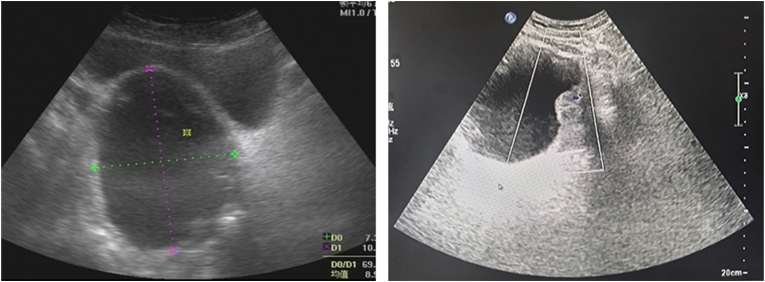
Urological ultrasound: A well-defined, anechoic lesion with clear borders is present posterior to the bladder. High-echoic nodules are visible on the cyst wall and colour Doppler flow imaging (CDFI) shows blood flow signals within the nodules.
